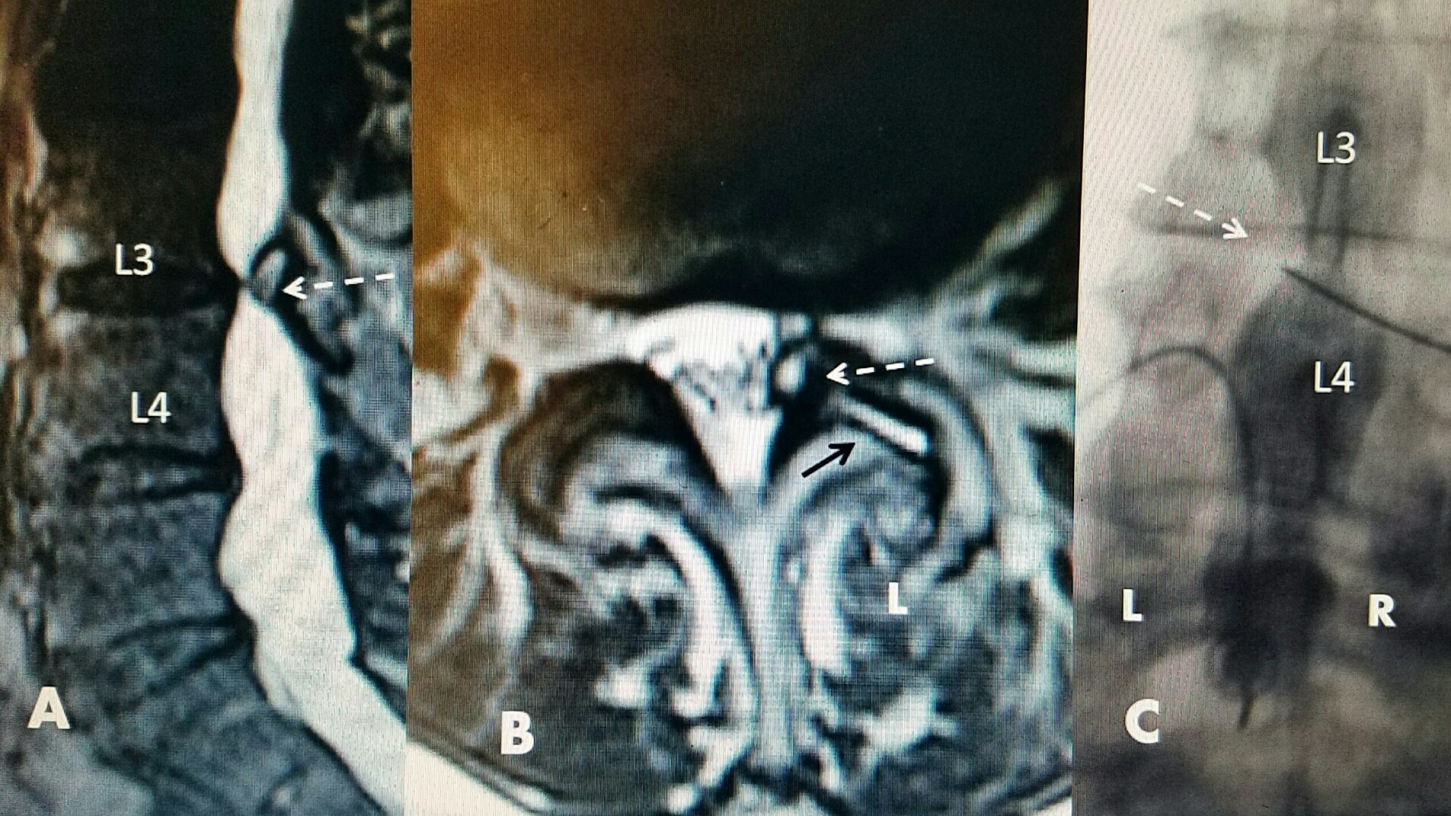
L3-4 facet cyst: magnetic resonance image (MRI) and intraoperative films A: Sagittal T2 spin MRI: localized L3-4 ventrolateral cyst compression (white dashed arrow) causing lateral recess stenosis. B: Axial T2 MRI: left (L) L3-4 facet cyst developing from the ventral surface of the facet joint with high T2 signal intensity (white dashed arrow) causing marked left foraminal stenosis with additional high signal intensity fluid in L3-4 facet joint (black solid arrow) on the same side as facet cyst (white dashed arrow). C: Intraoperative film: left (L) and right (R) are marked as seen in the prone position. Myelographic contrast outlining left L3-4 defect due to the facet cyst (white dashed arrow). The radiofrequency (RF) electrode is entering the defect obliquely through the interlaminar space.
